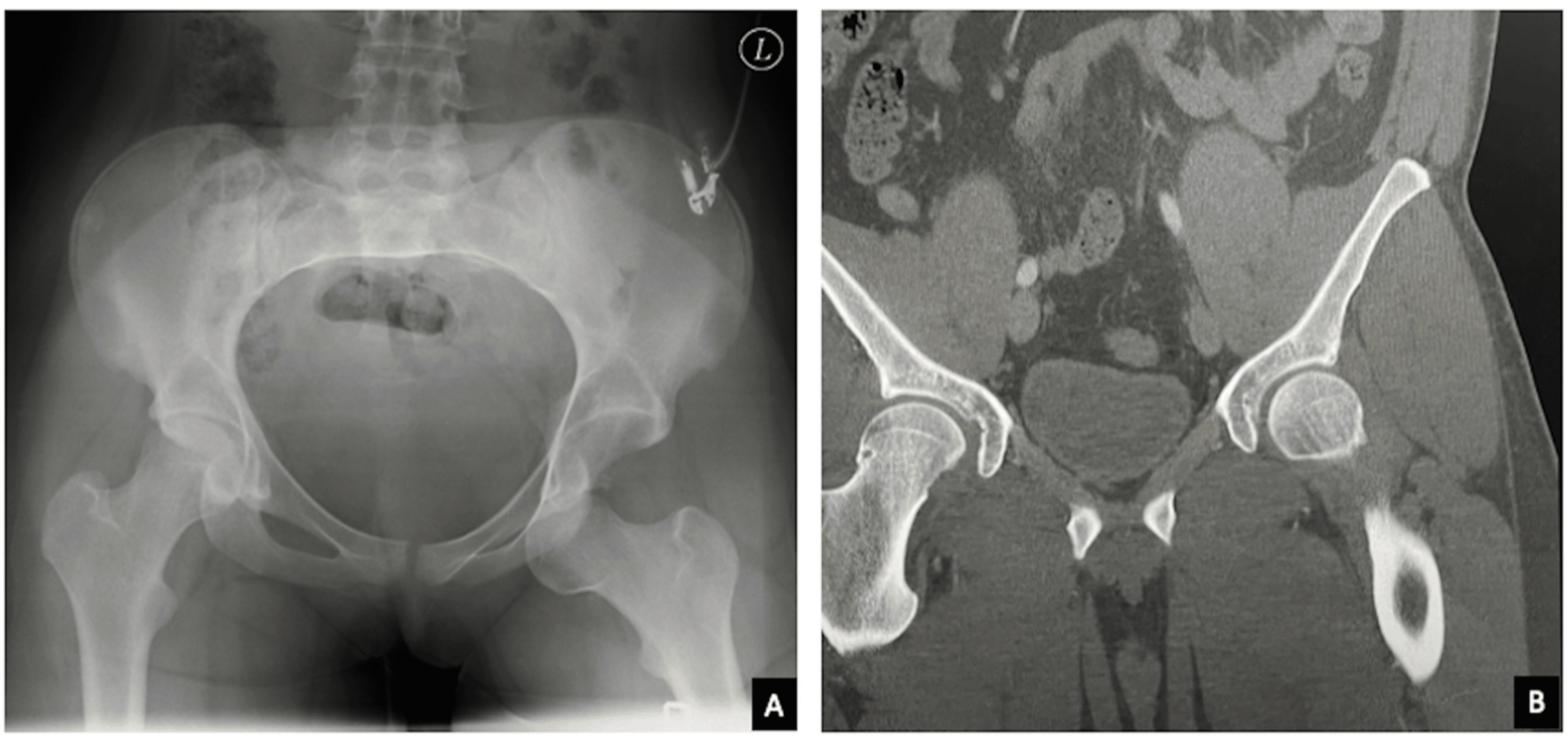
Radiographic image of a traumatic hip dislocation into the obturator foramen (A), and CT scan after closed reduction to detect concomitant acetabular or femoral head fractures, which were excluded in this case (B).
